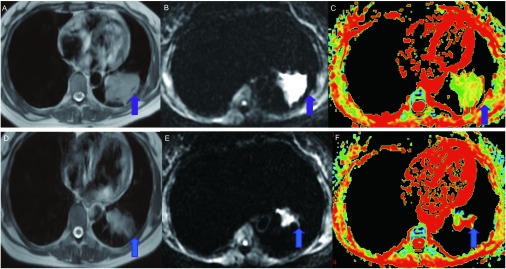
患者，女性，49岁，鳞癌。横轴位磁共振图，（化疗前）横轴位T_2_WI（A）、相应层面DWI图（B）及ADC图（C）。T_2_WI图左下叶不规则形长T_2_信号肿块，边缘不光整，DWI图上呈明显高信号（与邻近骨骼肌相比），ADC图以黄绿色区域为主，（箭头所示）；D-F（化疗1个月后）横轴位T_2_WI（D）、相应层面DWI图（E）及ADC图（F）。T_2_WI图肿块体积较前缩小，DWI图上呈高信号，ADC图以黄绿色伴红色区域为主，其中心部黄绿色区域范围较前减小（箭头所示）。DWI：磁共振扩散加权成像；ADC：表观扩散系数。 Transverse MRI images in a 49-year-old woman with squamous cell carcinoma of the lung (A, B, C: before chemotherapy; D, E, F: 1 month after chemotherapy). (A) T_2_-weighted MRI image showed an irregular hyperintense tumor (arrow); (B) On DWI image, the tumor was hyperintense in relation to adjacent skeletal muscle (arrow); (C) On ADC map, the tumor was depicted as an area of green and yellow(arrow); (D) T_2_-weighted MRI image showed that the size of tumor decreased (arrow); (E) On DWI images, the tumor was still hyperintense in relation to adjacent skeletal muscle (arrow); (F) On ADC map, the tumor was depicted an irregular contour with an area of green and yellow surrounding red. The area of color decreased (arrow). DWI: diffusion-weighted imaging; ADC: apparent diffusion coefficient.
